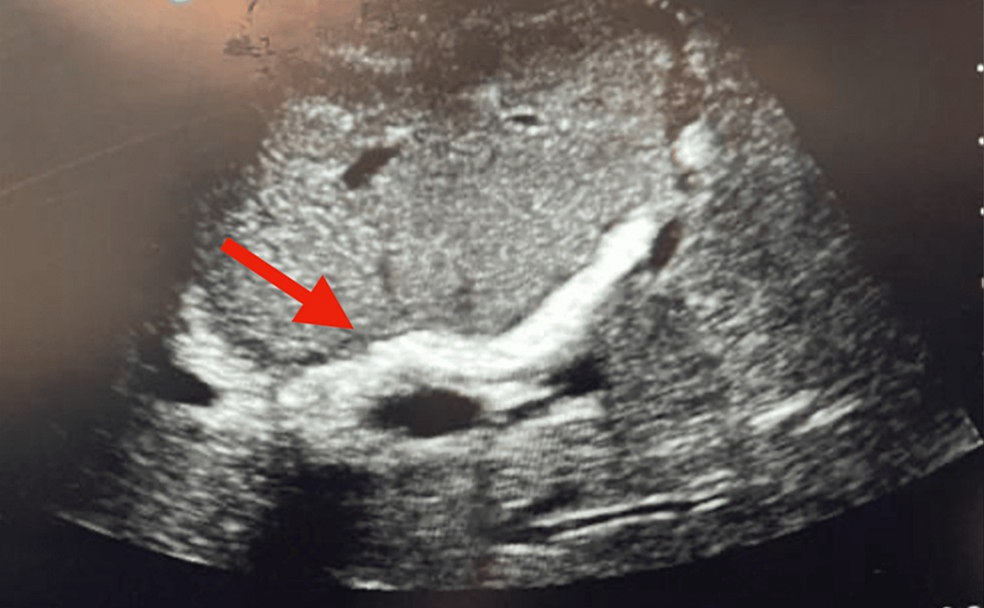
Symmers pipestem fibrosis Intraoperative ultrasonography revealed prominent portal spaces with surrounding hyperechoic areas (red arrow) reflective of Symmers pipestem fibrosis, a characteristic finding in advanced stages of hepatic schistosomiasis.
